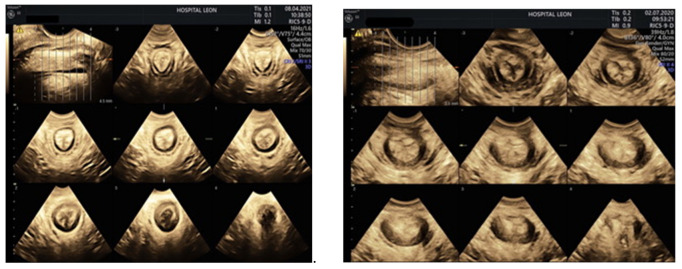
Tomographic ultrasound imaging of internal (IAS) and external anal sphincter (EAS). Longitudinal plane encompassing entire length of EAS is used as reference frame (upper left image of both pictures). The EAS appears as a ring of hyperechogenicity surrounding the thin hypoechogenic ring of the IAS. The mucosal folds of the anal canal appear in the center of the image as the innermost layer. Sphincters are normal in all the transverse slices of the left picture (complete rings). The picture on the right show a full-thickness defect of both sphincters (both concentric rings are interrupted).
